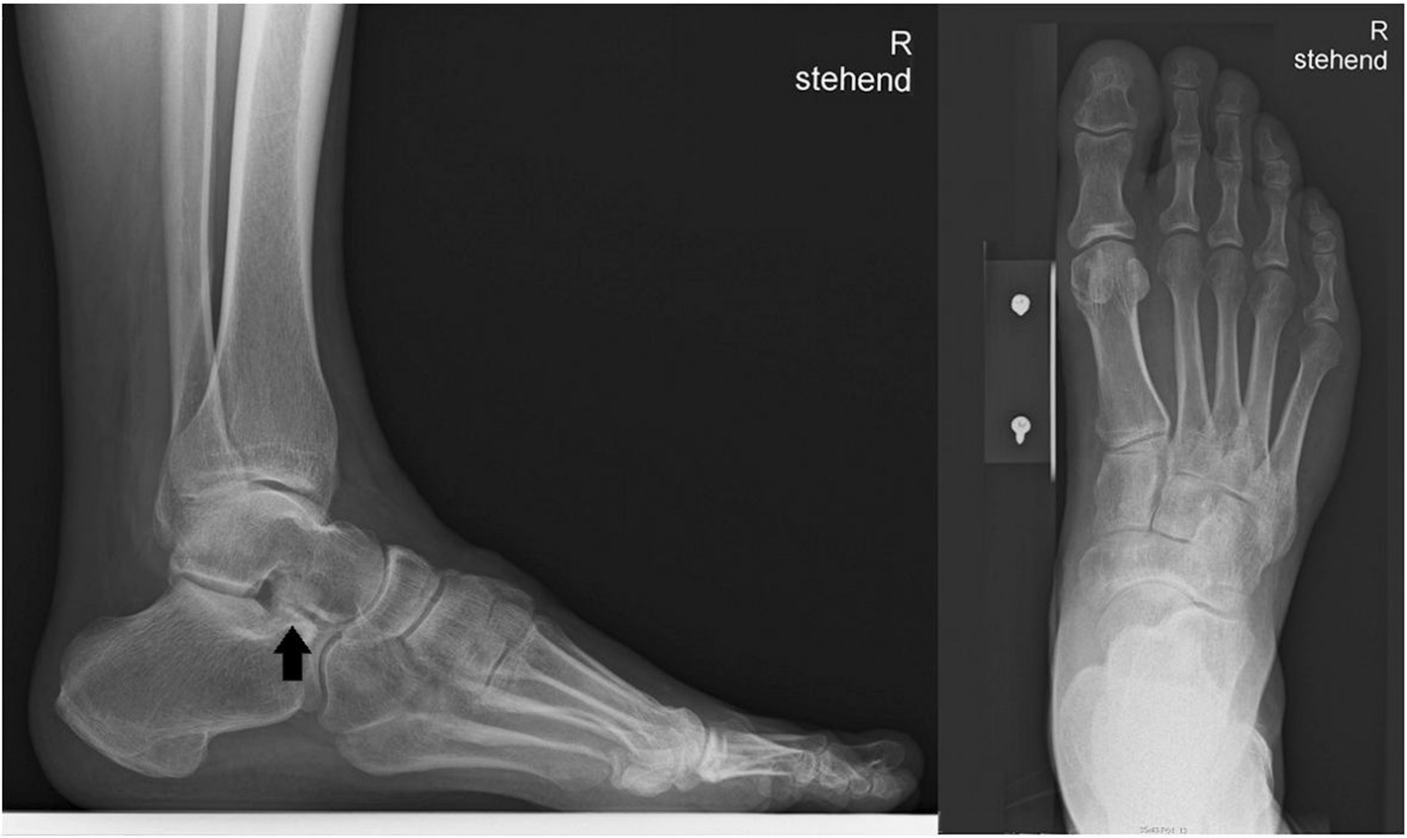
Lateral and dorsoplantar X-ray of the involved foot preoperatively. The arrow is pointing to the calcaneus secundarius.
